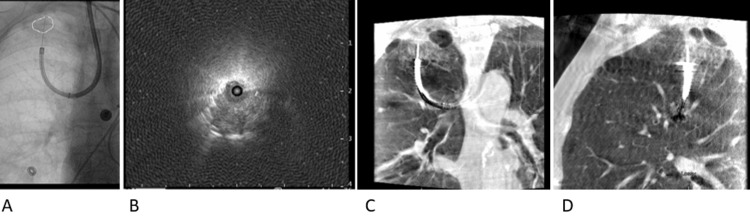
Augmented fluoroscopy to guide needle placement, brush placement, and transbronchial biopsies (A); Eccentric R-EBUS view of the lesion (B); Cone-beam CT coronal images (C) and sagittal images (D) were used to confirm the needle placement on the first pass.
